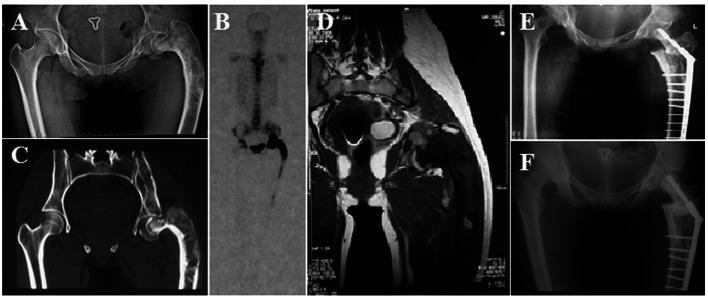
Polyostotic FD and shepherd’s crook deformity of a 28-year-old woman. (A) Anteroposterior preoperative radiograph; (B) preoperative radioisotope scanning; (C) preoperative CT; (D) preoperative MRI; (E) anteroposterior postoperative radiograph; (F) anteroposterior radiograph 4 years after sugery. FD, fibrous dysplasia.
